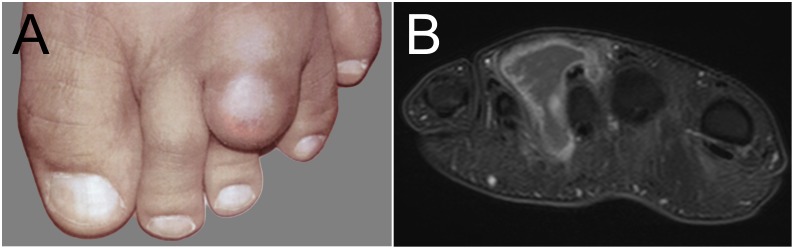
Case 1. Clinical presentation (A) before surgery: soft tissue swelling of the left forefoot. (B) T1-weighted fat-saturated post contrast MRI reveals a large polycyclic intermetatarsal mass surrounded by a thick wall.
